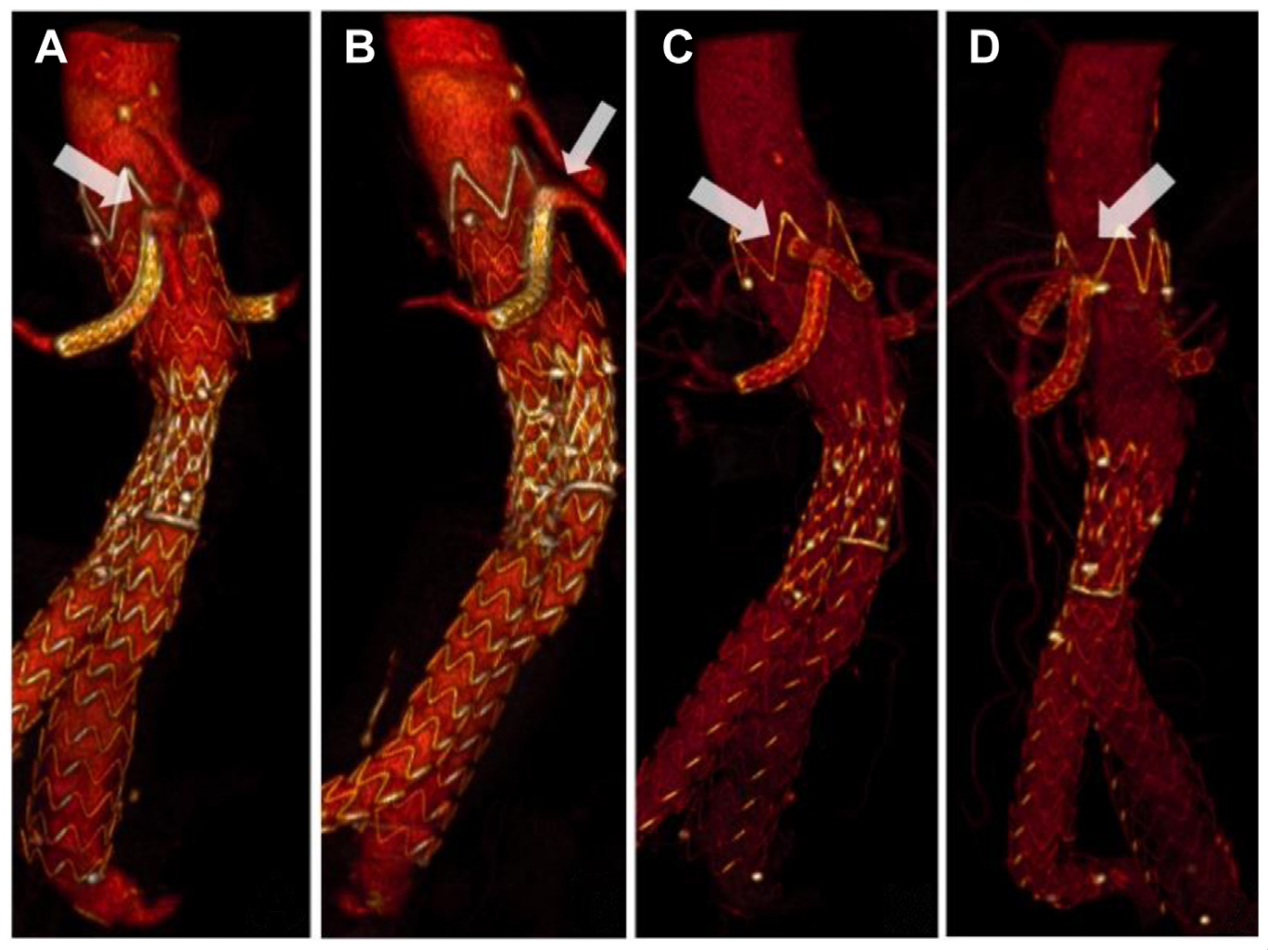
A,B, Preoperative three-dimensional reconstruction of computed tomography angiography (CTA). C,D, Postoperative three-dimensional reconstructions of CTA.
